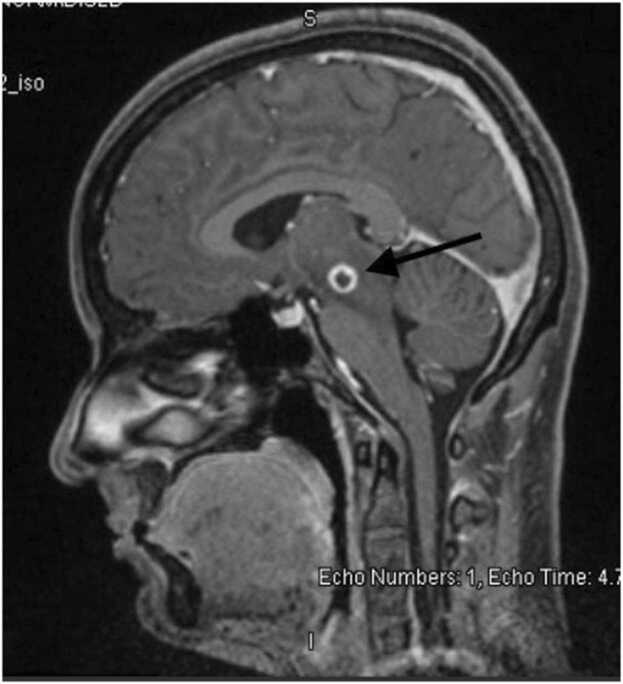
Sagittal view of MRI brain showing a midbrain ring-enhancing lesion 1.2 × 0.9 × 1 cm with central nodular calcification and significant peri-lesional edema (black arrow).
